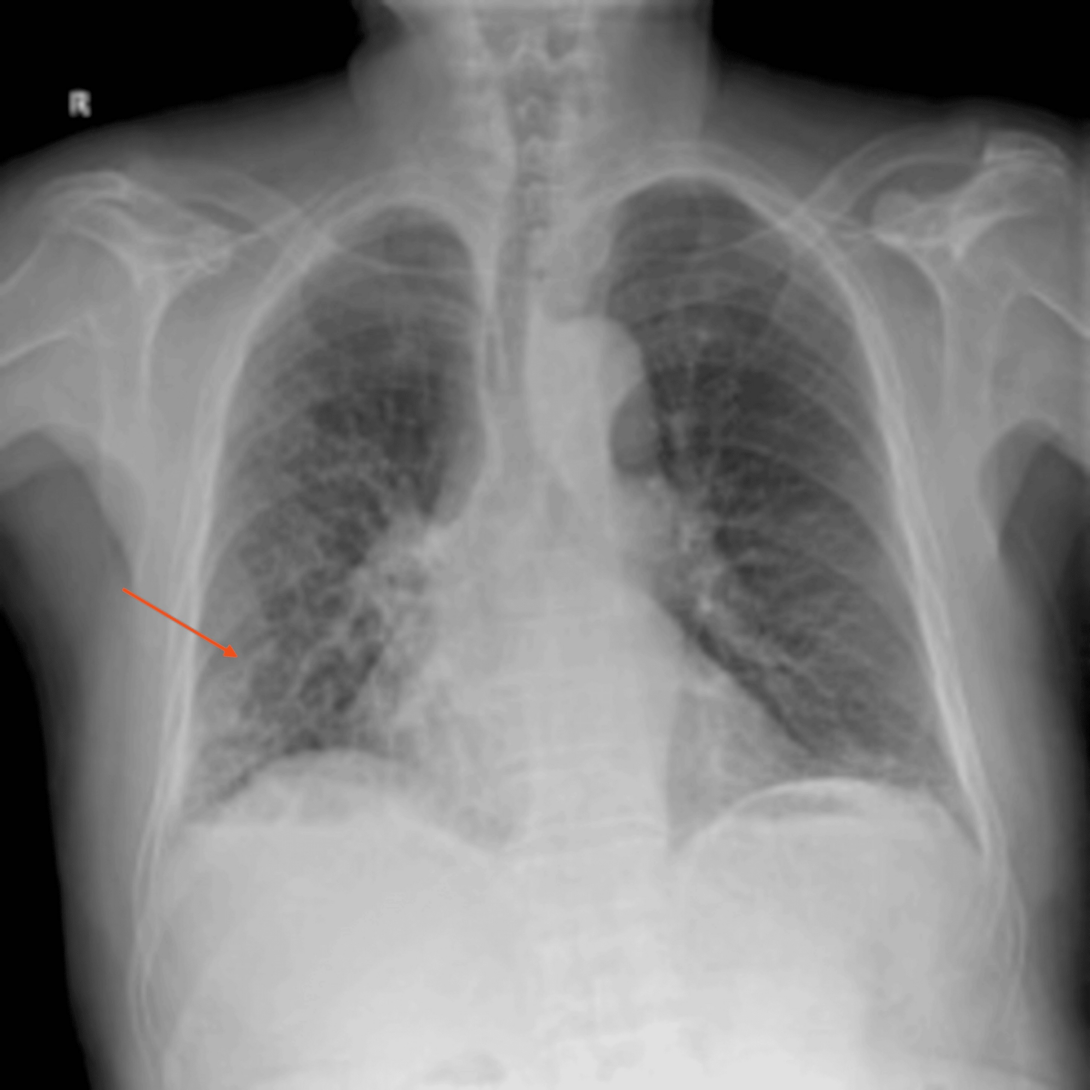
Post-admission chest X-ray showing increased opacity in the right lower lung field, indicated by the red arrow. Chest X-ray revealing diffuse aspiration bronchiolitis: red arrow (RLLF consolidation); additional multilobar findings (tracheal wall sputum, left lower lobe infiltrate, right upper/middle infiltrates) - characteristic of chronic silent aspiration in frail elderly. RLLF: right lower lung field
